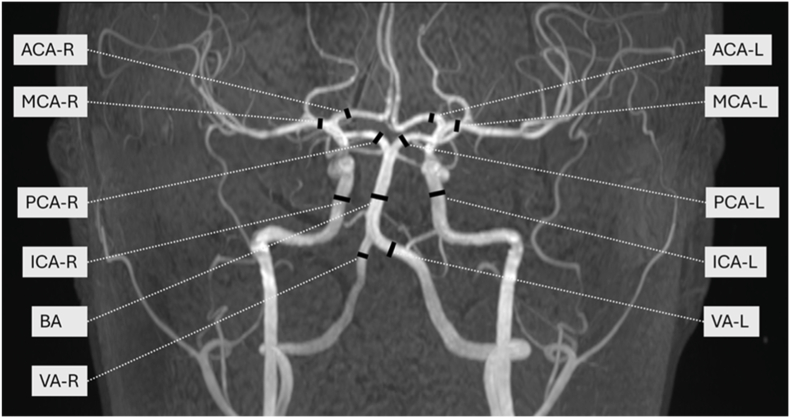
TOF-MRA of the intracranial arteries with marked locations for intracranial flow measurements with the RT-PC MRI. Measurement locations are marked as left (L) or right (R) site. MCA: medial cerebral artery; ACA: anterior cerebral artery; PCA: posterior cerebral artery; BA: basilar artery; VA: vertebral artery; ICA: intracranial carotid artery.
